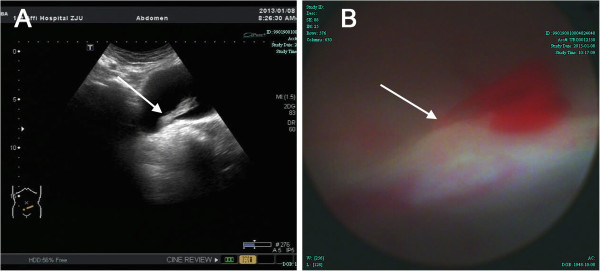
Ultrasonography and cystoscopic finding of a woman with an 8-day history of painless gross hematuria. (A) Ultrasonography revealed a long strip, 4.2x1.2 cm sized, hypoechogenic mass (*arrow*) floating in the bladder, its pedicle located at the bladder base. (B) Cystoscopic examination revealed a pulsatile hemorrhage site (*arrow*) from the right bladder base.
